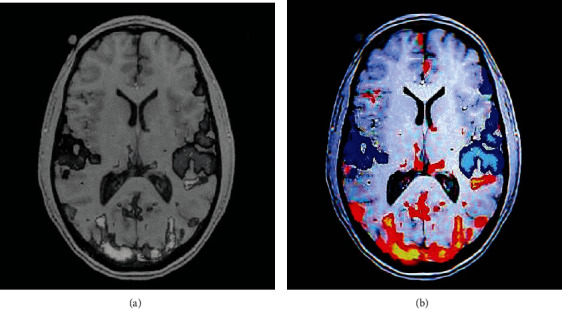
MRI scan of a patient's brain. (a) was a normal MR scan; (b) was an MRI scan after k-means clustering.
